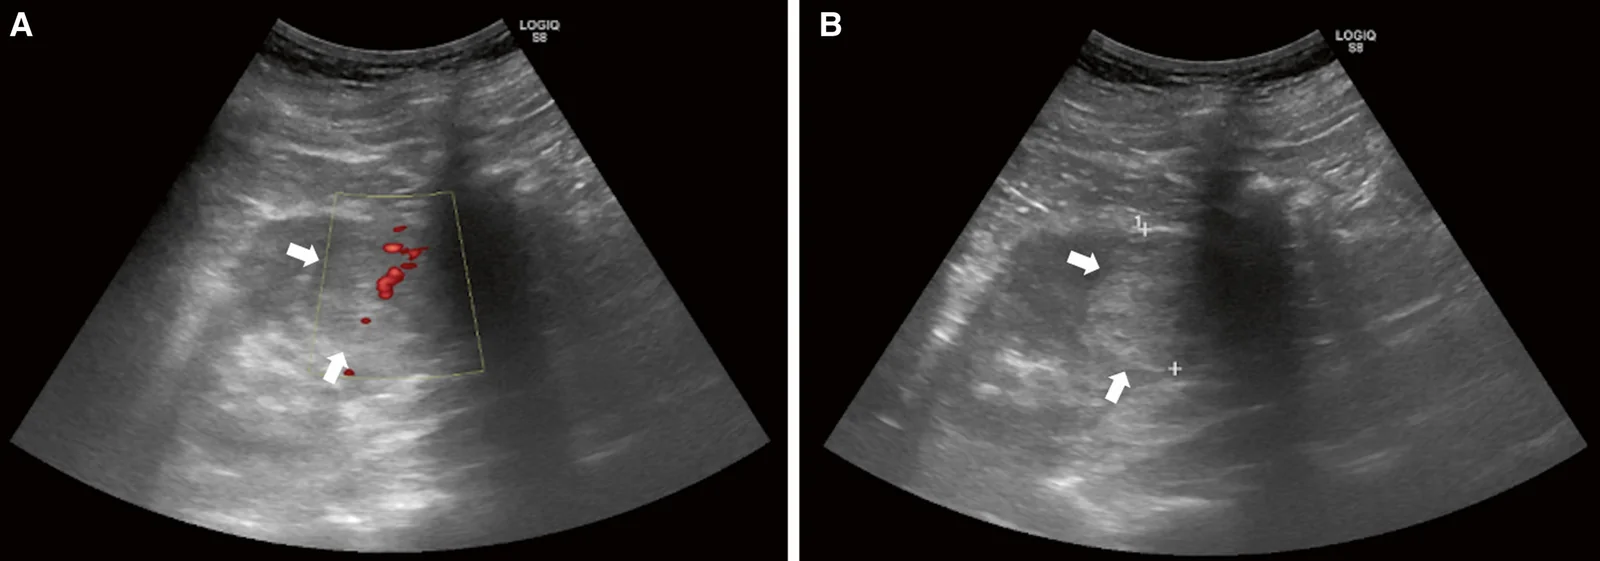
Abdominal ultrasound images showing the presence of vascular flow within an isoechogenic mass at the lower pole of left kidney using colour Doppler (*A*, arrows), and an isoechogenic mass without colour Doppler (*B*, arrows).
